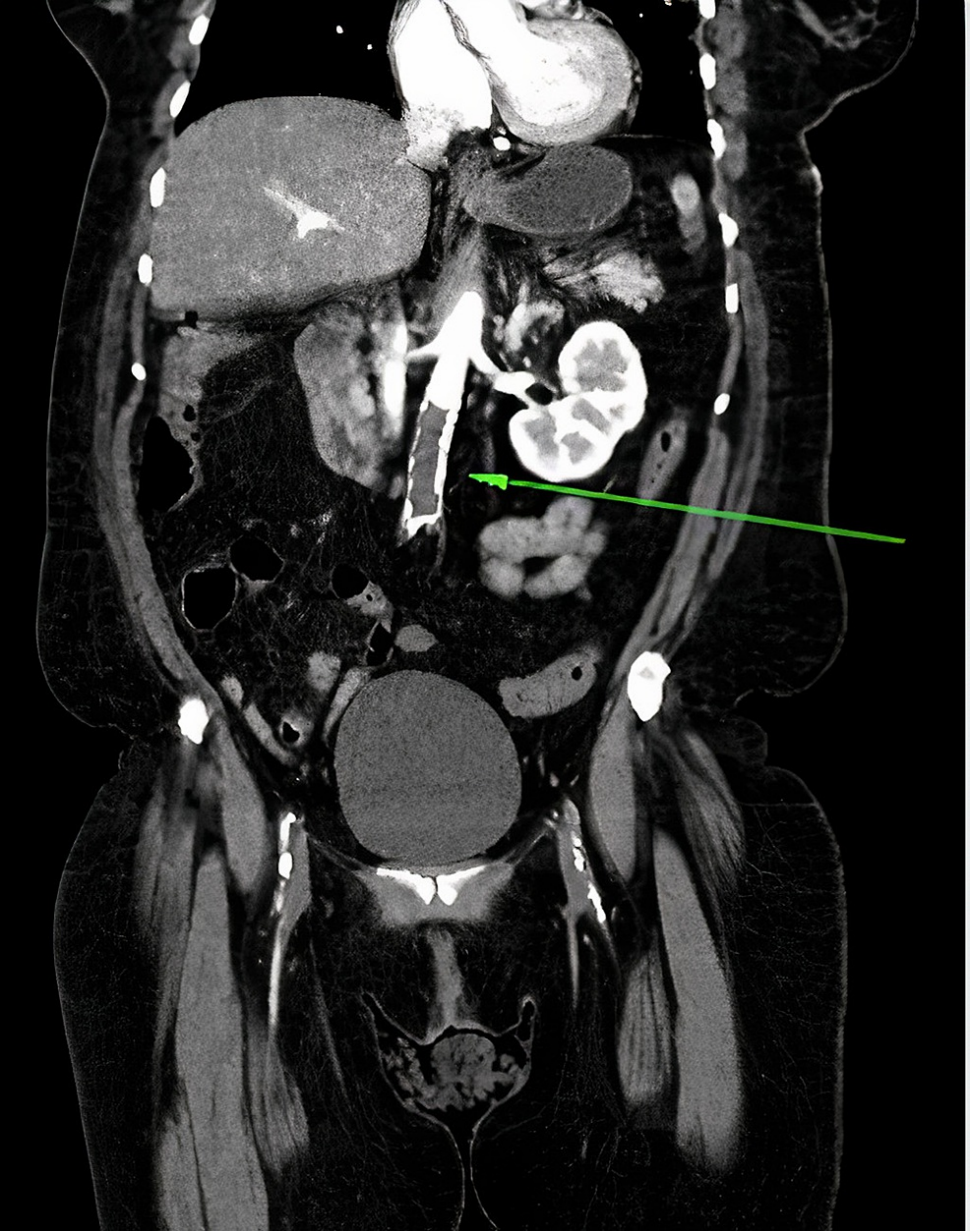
CT abdomen and pelvis with contrast demonstrating a large filling defect inferior to the renal arteries which extends to the level of the proximal superficial femoral artery bilaterally (Green Arrow). Image courtesy of Kristian O. Loveridge, D.O.
